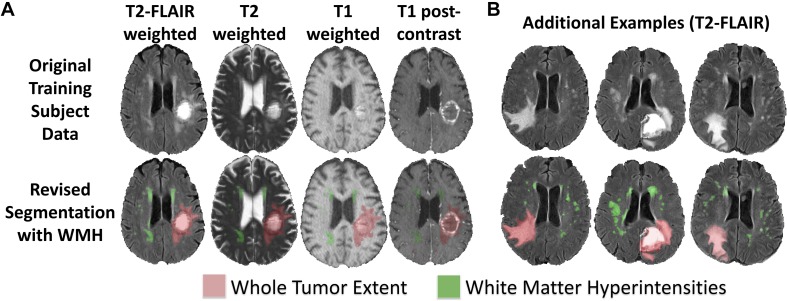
Revised BraTS 2018 training segmentations including annotations of WMH. (A) Sample revised segmentation of abnormal signal due to WT (red) and WMH (green) on T2-FLAIR, T2, T1, and T1PC axial slices. (B) Three additional example revised segmentation maps for tumor and WMH overlaid on T2-FLAIR axial slices.
